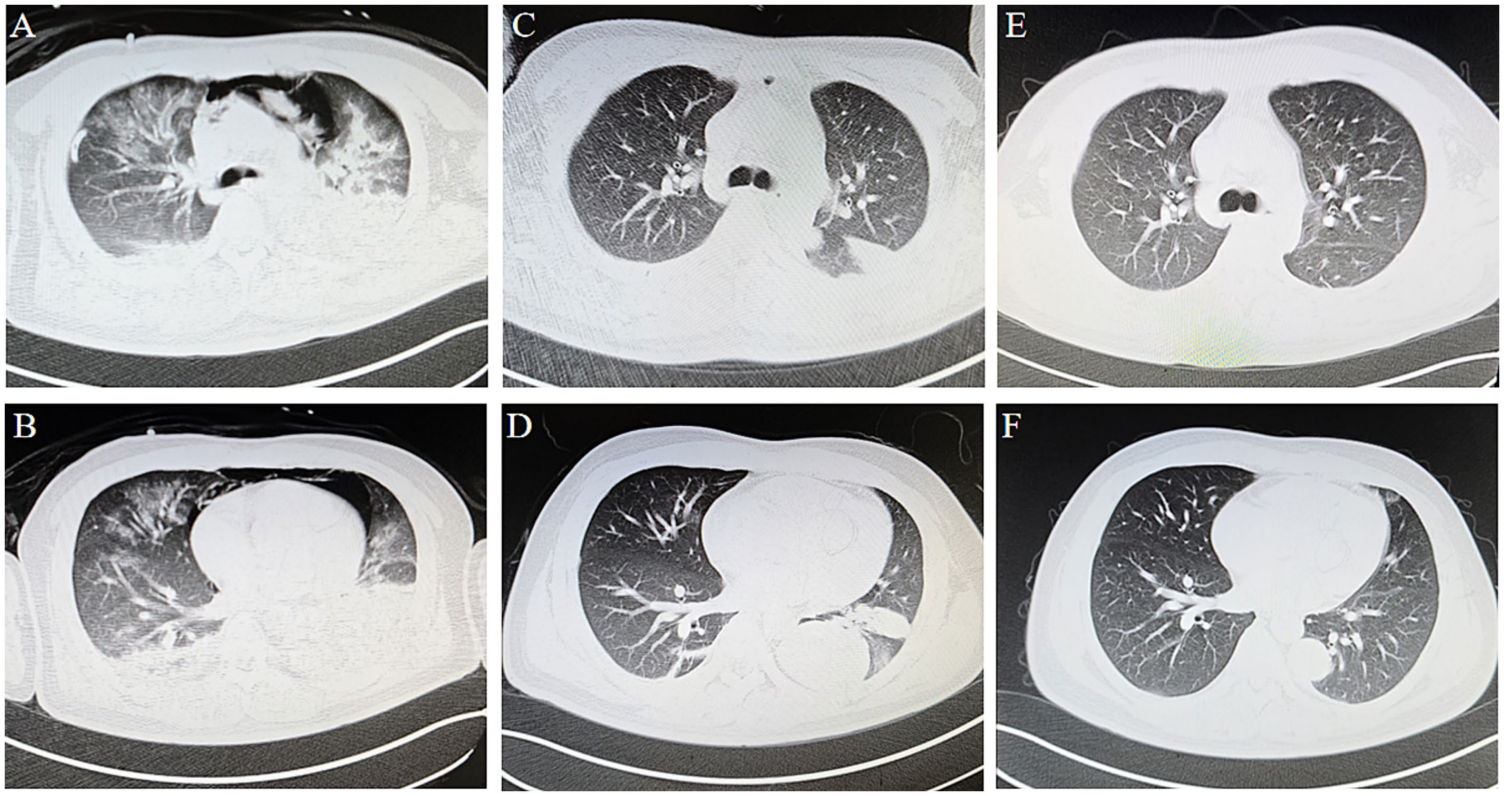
Thoracic CT. (A,B) At 72 h of ECMO, CT demonstrating bilateral pulmonary edema with extensive ground-glass opacities, patchy consolidations and pneumomediastinum. (C,D) Thoracic imaging demonstrated complete resolution of pulmonary edema, with residual consolidative opacities persisting in bilateral lower lobes. (E,F) The 3-month follow-up showed that the patchy shadows were significantly reduced, and had basically recovered.
